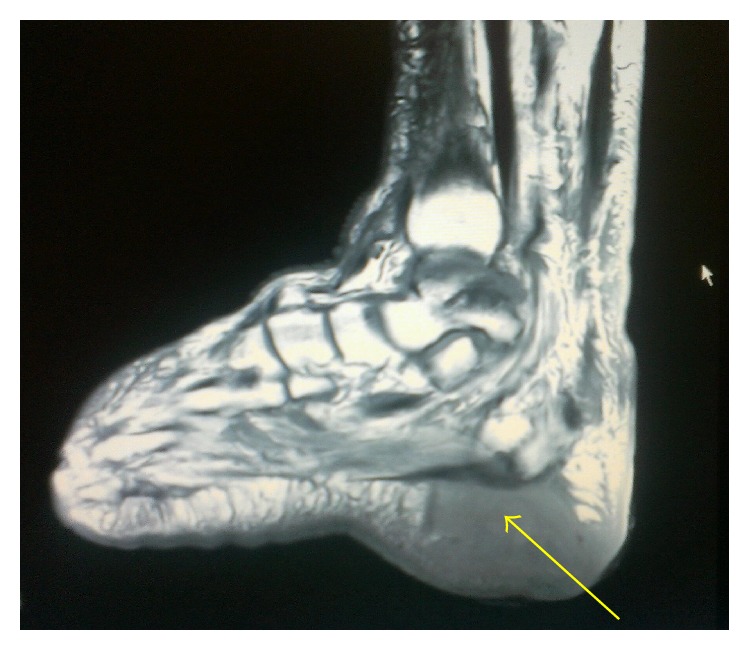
The MRI showed a destructive lesion in posterior part of calcaneus.
